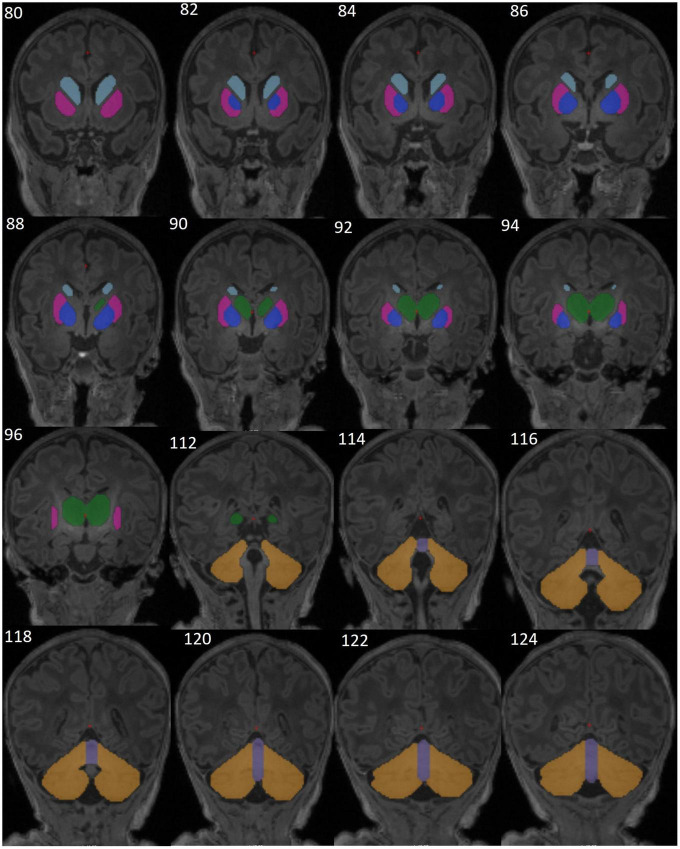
Representative T1-weighted brain MR images from one neonate without HIV exposure or infection (HUU), showing the manually segmented structures of the basal ganglia [caudate (sky blue), putamen (magenta), pallidum (dark blue)], thalamus (green), cerebellar hemispheres (yellow), and cerebellar vermis (purple). Images are in coronal view (slices 80-96 and 112-124).
